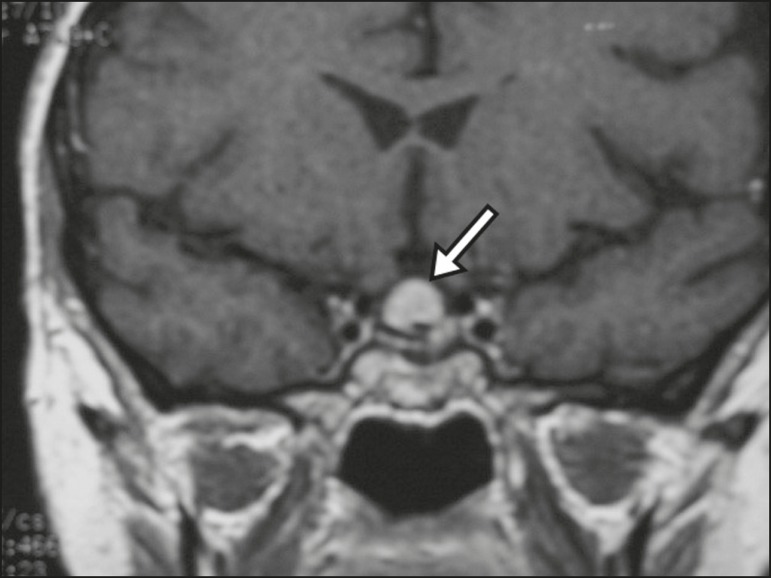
Rathke’s cleft cyst. Coronal T2-weighted MRI sequence showing an
